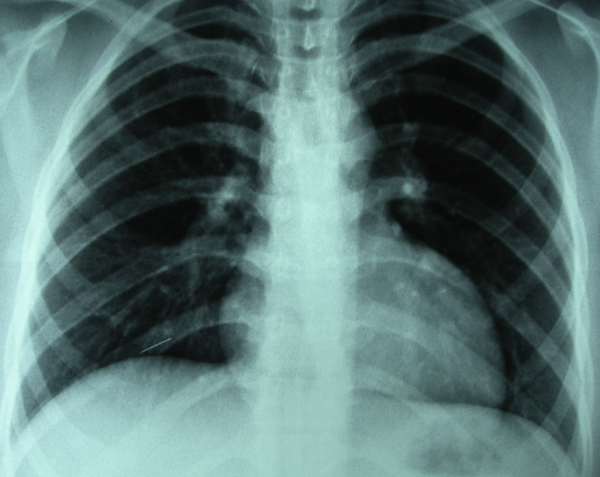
Chest roentgenogram showing the needle in the right cardiophrenic sinus.
